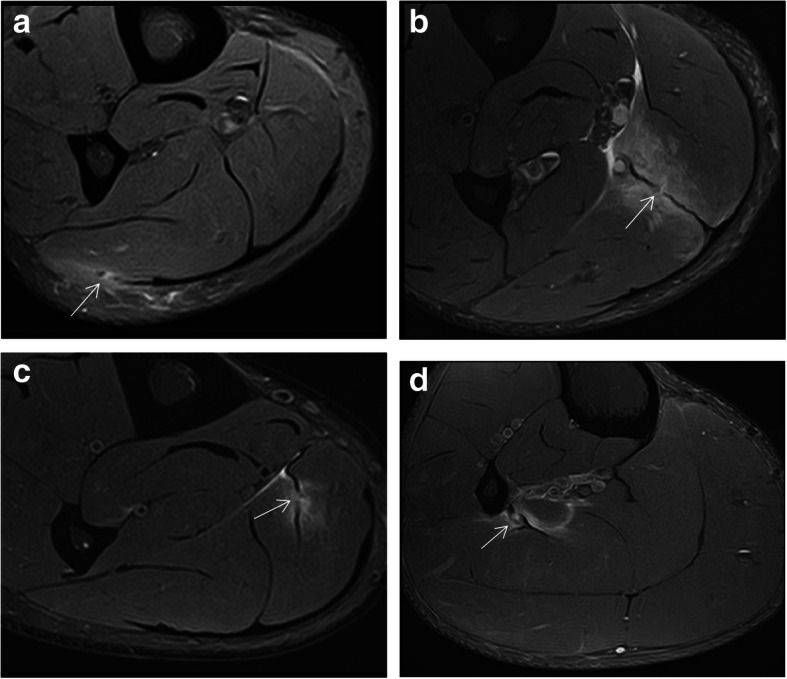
In the soleus, axial MR images in fluid-sensitive sequences of four different patients show edema surrounding the connective tissue injured and its discontinuity (arrows) involving different structures: (a) the posterior aponeurosis, (b) central septum, (c) medial fascicle, and (d) lateral fascicle
